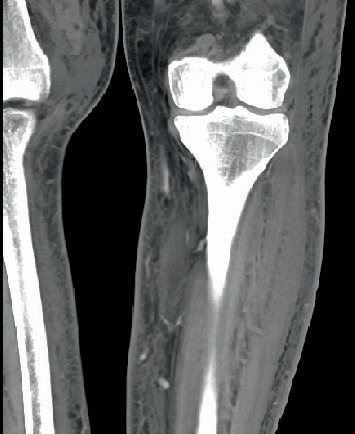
CT scan of the left lower extremity showing soft tissue swelling (blue arrows).
